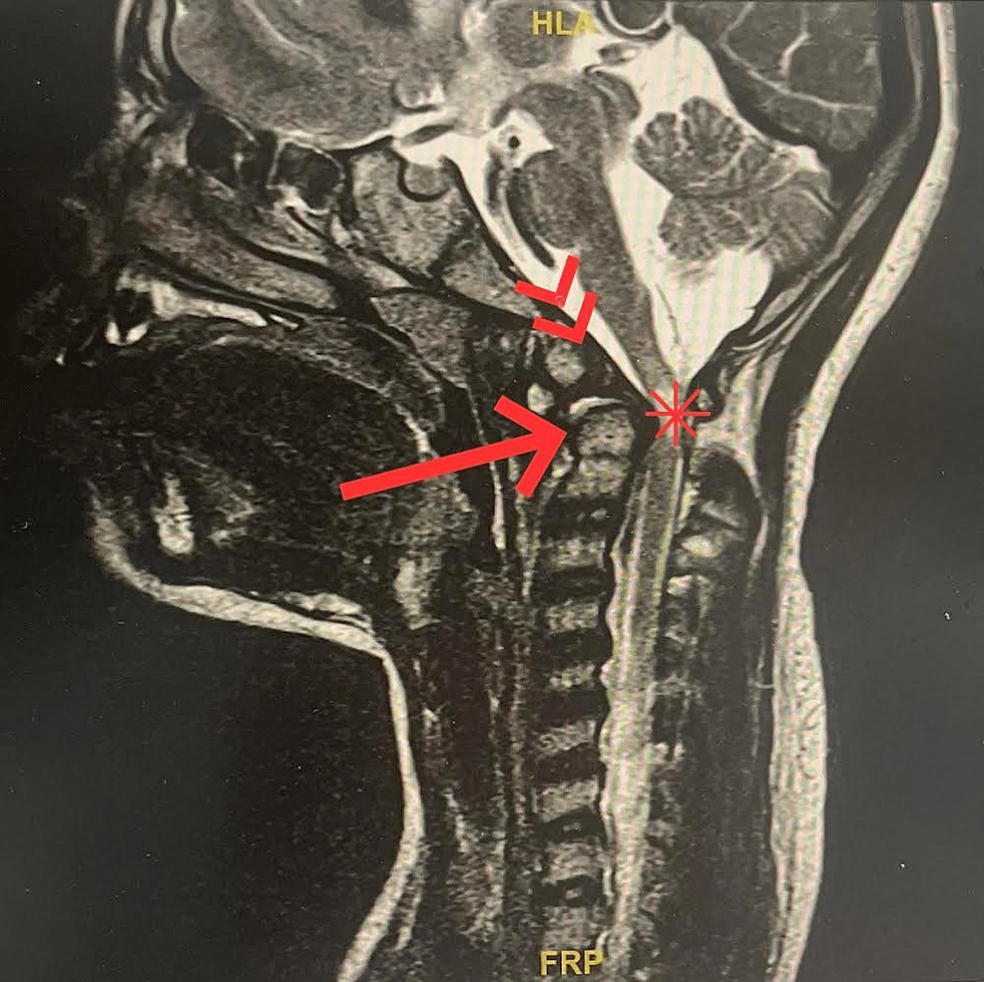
Brain MRI Normal variant Os odontoid (double arrow) superimposed with a retroverted odontoid process (arrow) results in focal compression of the upper cervical cord and subsequent chronic myelopathy (asterisk).
